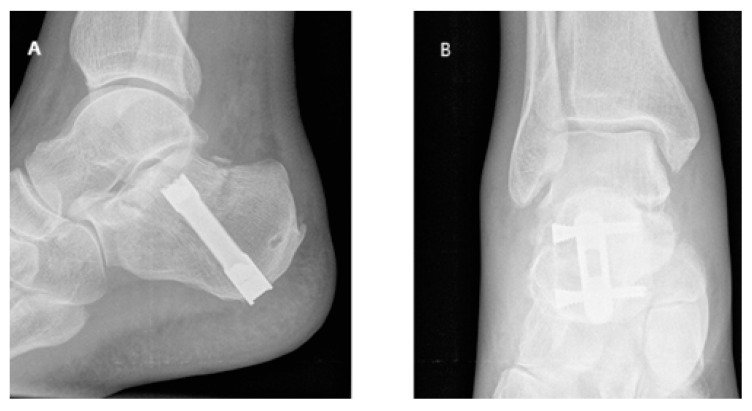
CRIF with CALCAnail^®^: (A) lateral and (B) anterior–posterior (AP) radiographs.
